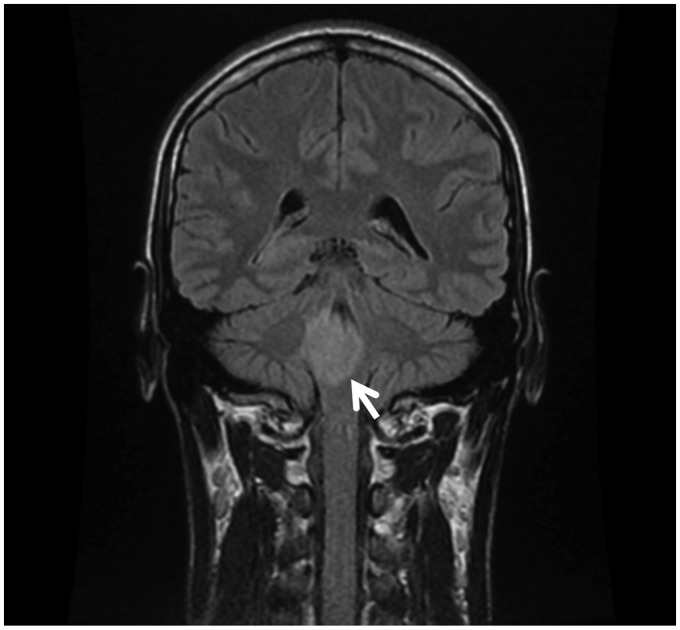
MRI brain showing a large ring enhancing lesion of the brainstem (arrow).
